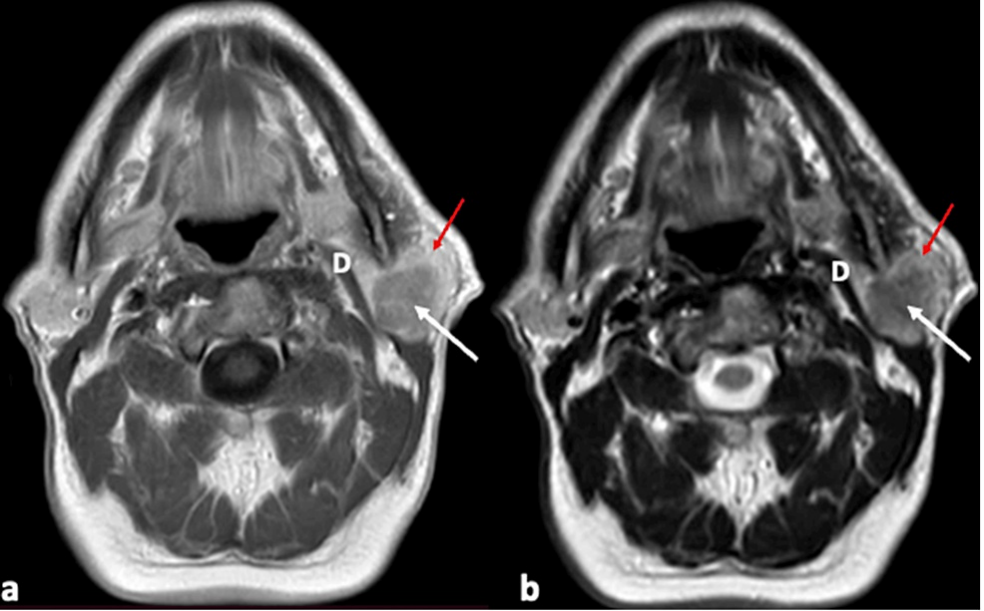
MRI, axial view: (a) T1-weighted and (b) T2-weighted. Parotid gland (red arrow); tumor (white arrow) MRI, magnetic resonance imaging; D, digastric muscle
